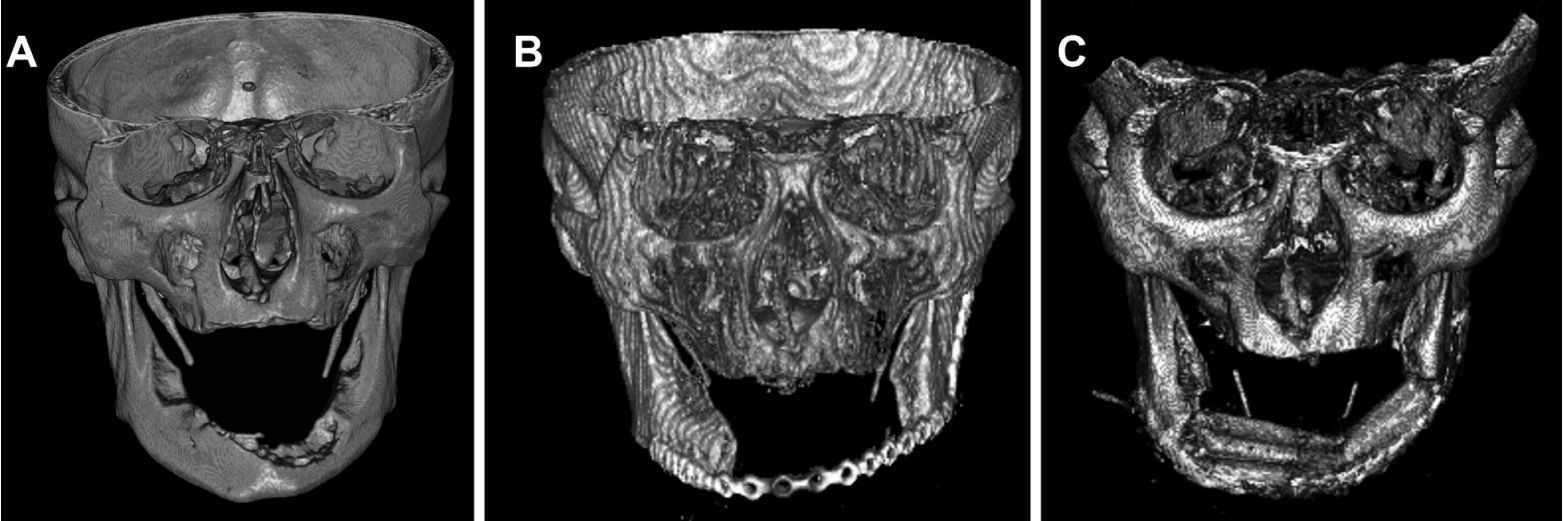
A: 3D-CT image before tumor resection. The infiltration of the bone is clearly visible. B: 3D-CT image after tumor resection with a mandibular continuity defect. C: 3D-CBCT after fibular osseomusculocutaneous flap reconstruction.
